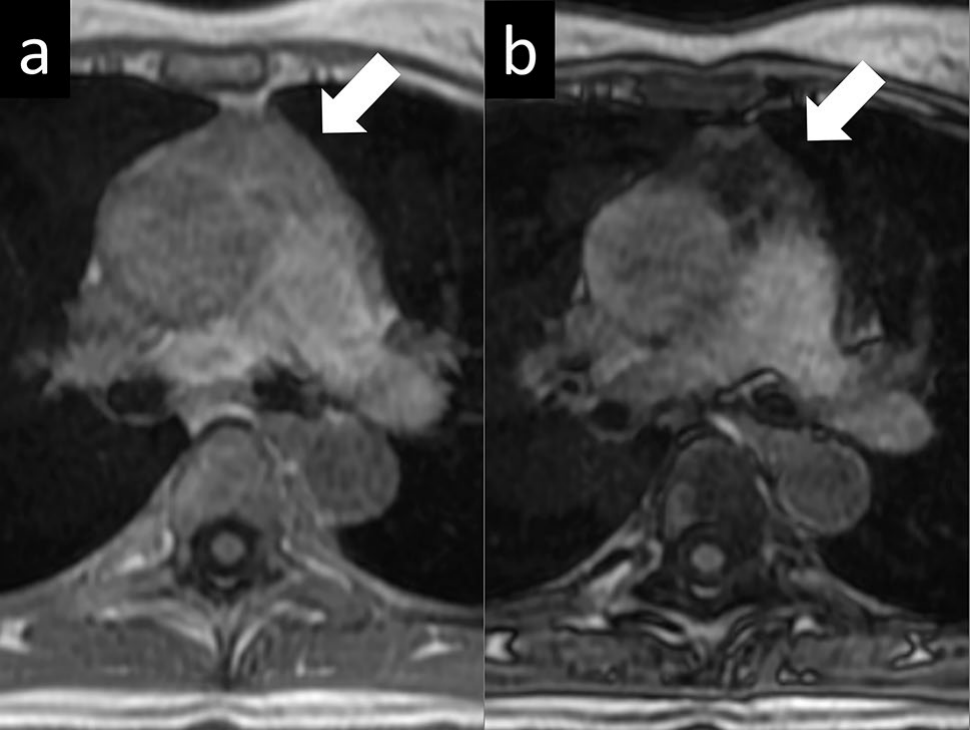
Thymic hyperplasia. A 40-year-old woman with thymic hyperplasia. Transverse in-phase a and opposed-phase b gradient-echo T1-weighted MR images indicate an apparent decrease in the signal intensity of the thymus on the opposed-phase image relative to the in-phase image (arrows). The CSR is 0.45. MR, magnetic resonance; CSR, chemical shift ratio
